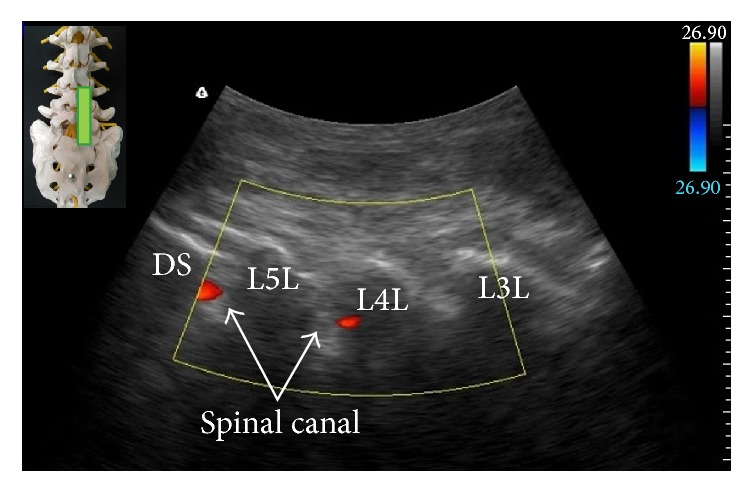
Color Doppler ultrasonography in paramedian sagittal oblique view of the sacral and lumbar spine. The observed color spectrum suggests the flow of injectate reaching L4-5 level. The inset shows the position of the ultrasound transducer. L3L: L3 lamina; L4L: L4 lamina; L5L: L5 lamina; DS: dorsal surface of sacrum.
